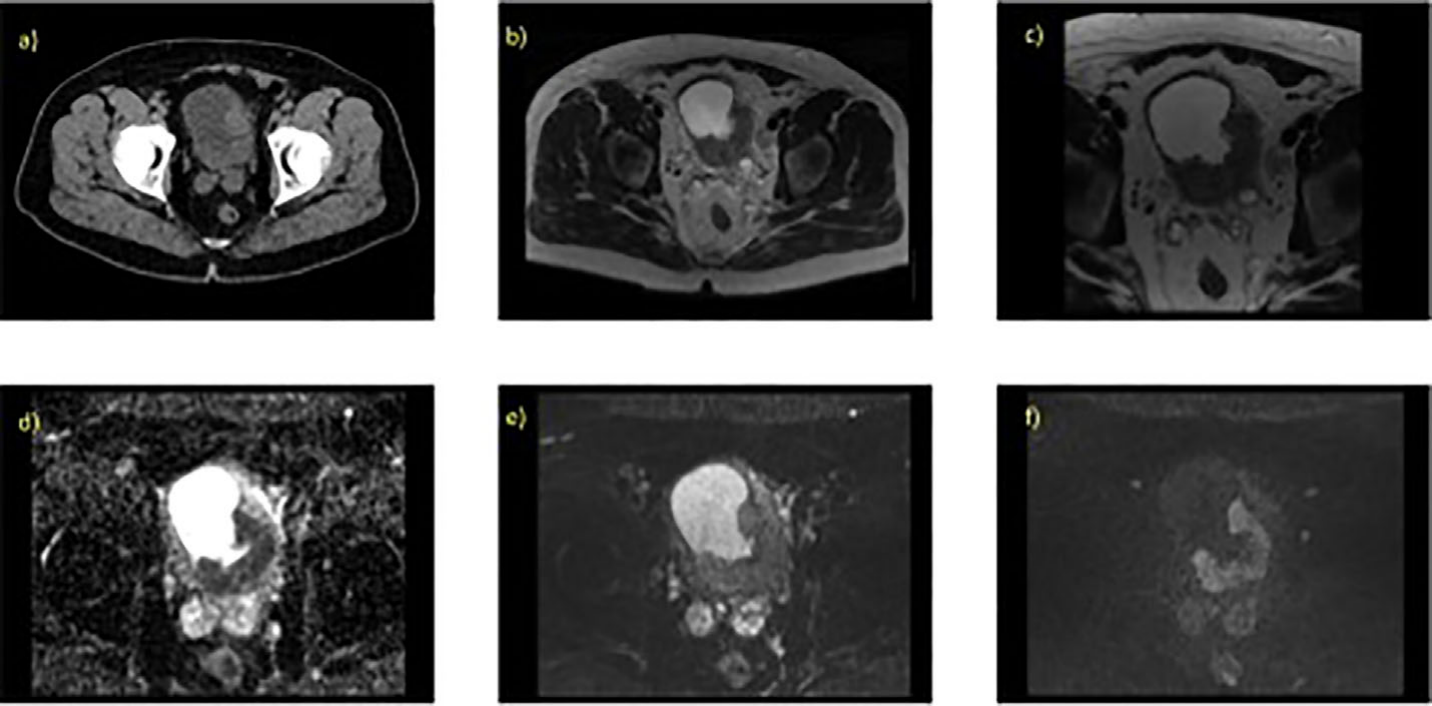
Localized MIBC as evaluated on T2W and DWI with the associated parameter settings for 1.5T MRI. 70 year old male with known T3 N0 M0 bladder cancer, tumour is present at the left ureteric orifice (extending posteriorlaterally) (A) contrast enhanced CT scan, axial slice through pelvis, (B) axial T2W (large field of view) showing hypo intense lesion, (C) axial T2W small field of view (D) corresponding ADC map, (E) axial DWI at b-value 0, (F) axial DWI at b-value 750.
